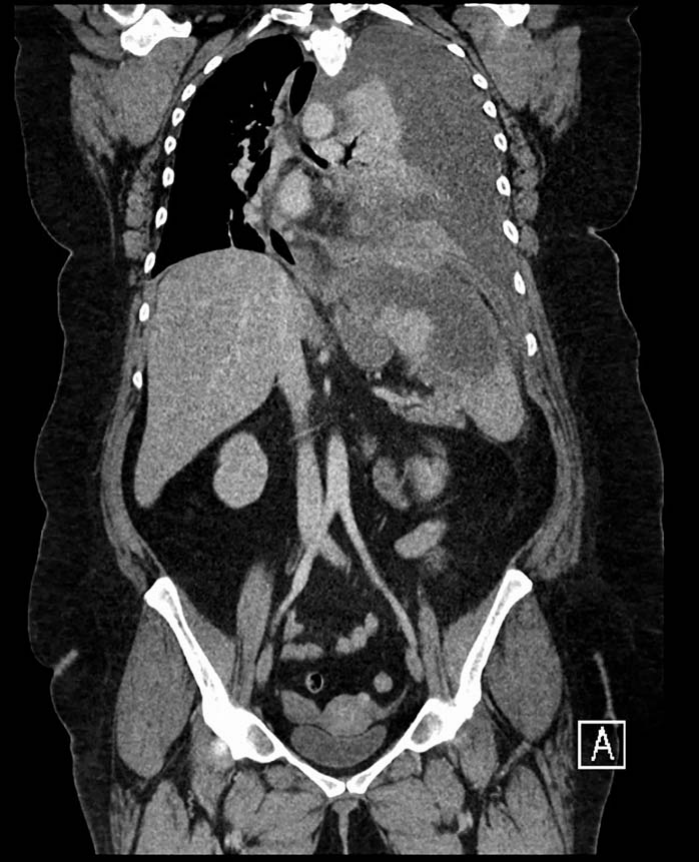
CT chest, abdomen and pelvis coronal view: left-sided empyema and splenic abscess.
